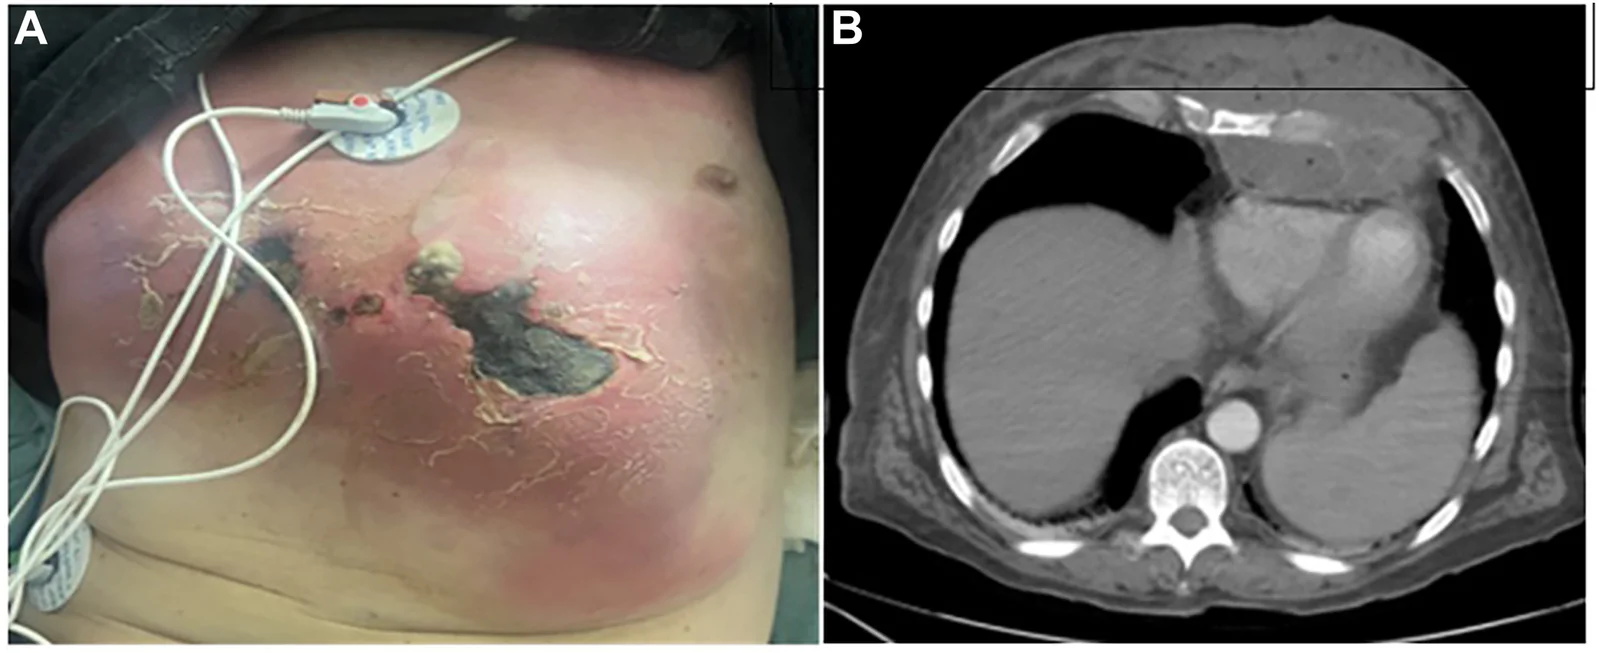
(A) Admission photograph of an extensive chest wall soft tissue infection with necrosis of skin over an open costal arch fracture. (B) Axial computed tomographic scan showing an abscess of the anterior chest wall and a soft tissue infection consistent with necrotizing soft tissue infection.
